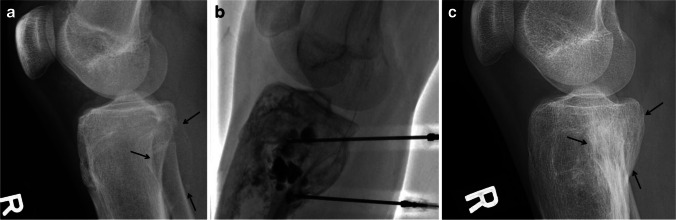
Type III aneurysmal bone cyst in a 16-year-old boy before, during and after percutaneous sclerotherapy with doxycycline. a A lateral radiograph of the proximal tibia shows an eccentric, well-defined, lytic and expansile lesion (*arrows*) involving the proximal tibial metaphysis with a faint peripheral calcified shell. b A fluoroscopic image of the lesion during sclerotherapy shows the insertion of two spinal needles and injection of contrast accumulating in several intralesional locules. c A lateral radiograph of the proximal tibia 6 years later after several treatments with sclerotherapy shows complete interval healing of the lesion (*arrows*) with some sclerosis and remodeling of the bone but no significant residual deformity
